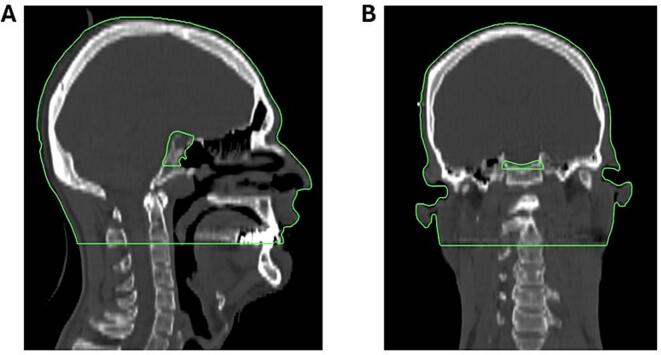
Head minus CTV (Head‐CTV) is defined as the external contour (excluding fixation equipment) cranially from the top of the skull to the caudal dorsal border of dens axis (C2), excluding the CTV itself. Fig. 1 displays an example of Head-CTV (green contour) for a patient with a pituitary neuroendocrine tumor (PitNET), CT dose plan is shown in bone window.
